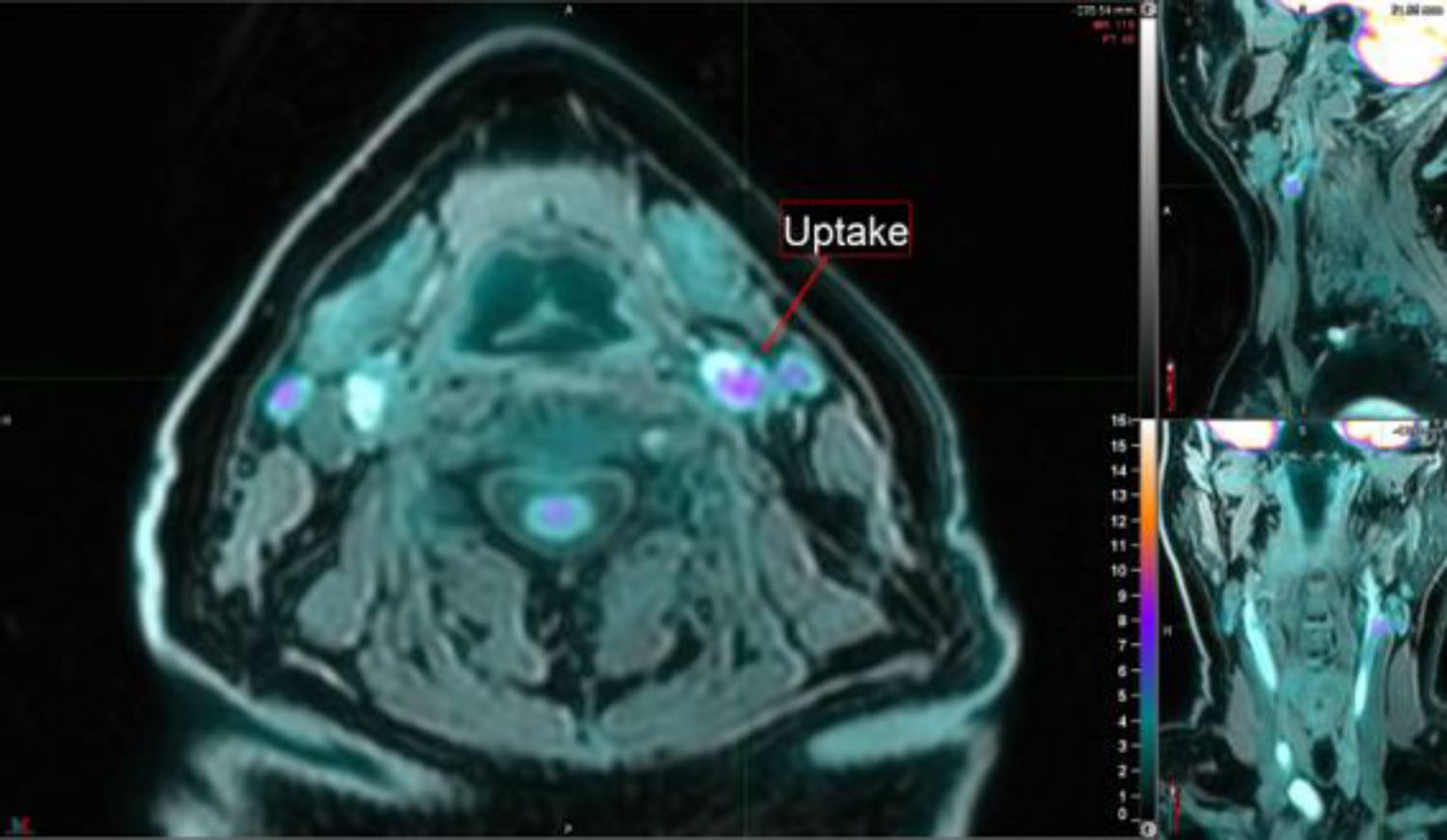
Increased ^18^F-FDG uptake in a carotid plaque (shown by the red arrow) in fusion images consisting of T1W MRI and PET. The anatomy of the neck, including the carotid arteries is presented in the axial- (left), sagittal- (upper right) as well as the coronal (lower right) planes. The image was created using MIM, MIM Software version 6.9.3 (MIM Software Inc. Cleveland, OH, USA, www.mimsoftware.com.
